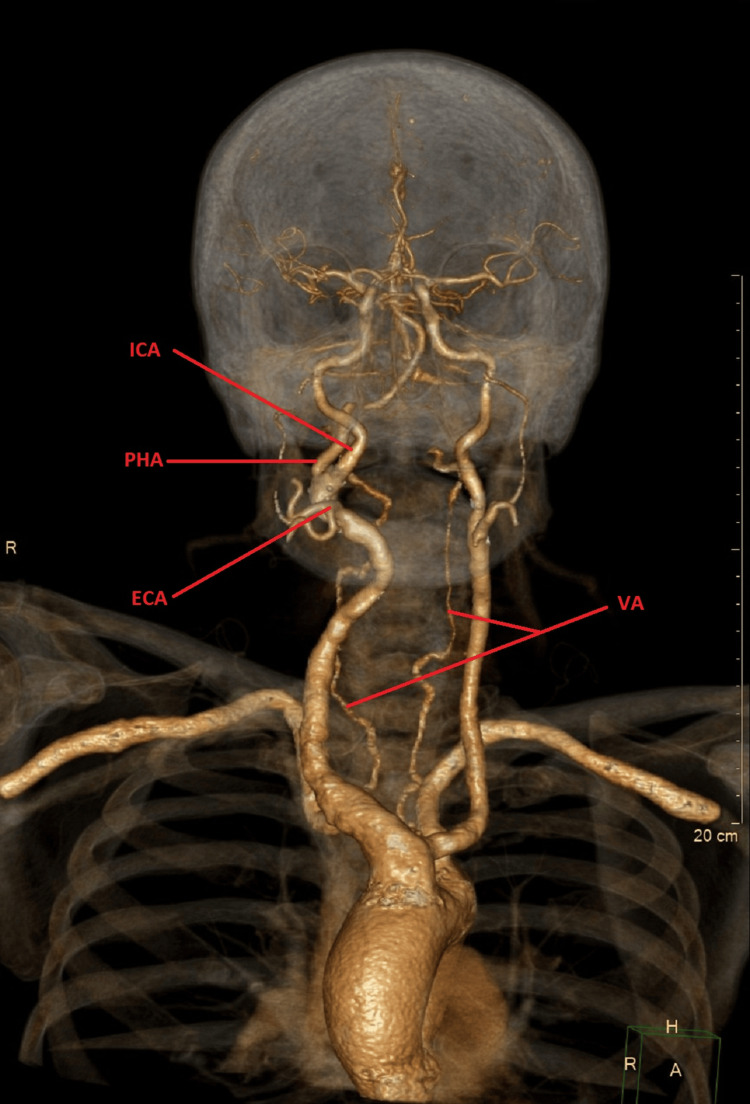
The origin of the PHA from the cervical segment of the internal carotid artery is associated with hypoplastic vertebral arteries in the cervical segment. MSCT angiography of supra-aortic branches in a 57-year-old female patient reveals the origin of persistent hypoglossal artery from the cervical segment of the internal carotid artery. The image shows hypoplasia of both vertebral arteries. MSCT: Multi-slice computed tomography; ICA: Internal carotid artery; ECA: External carotid artery; PHA: Persistent hypoglossal artery; VAs: Vertebral arteries
